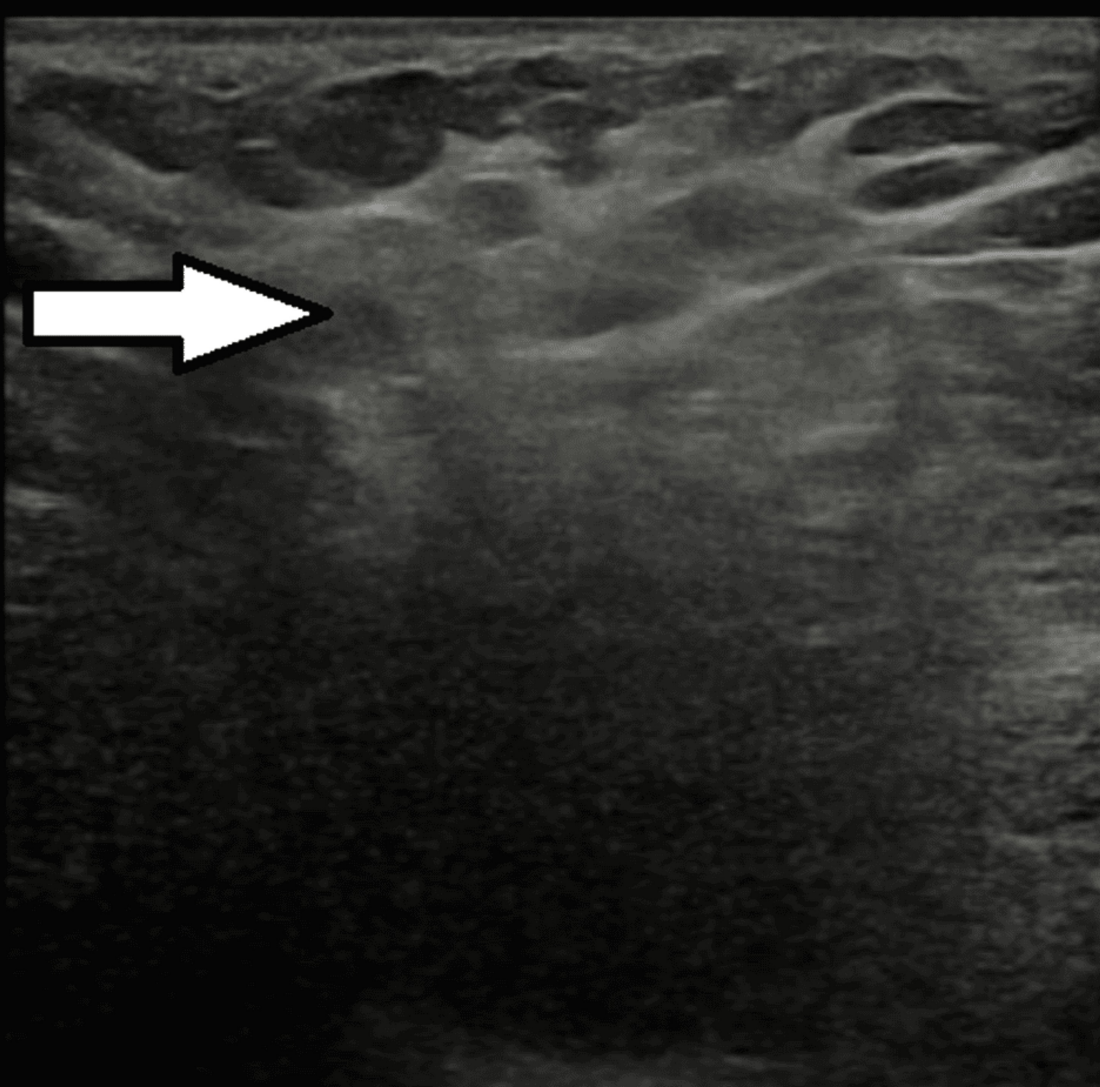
Repeat ultrasound after one week demonstrating a deep-seated abscess (arrow).
